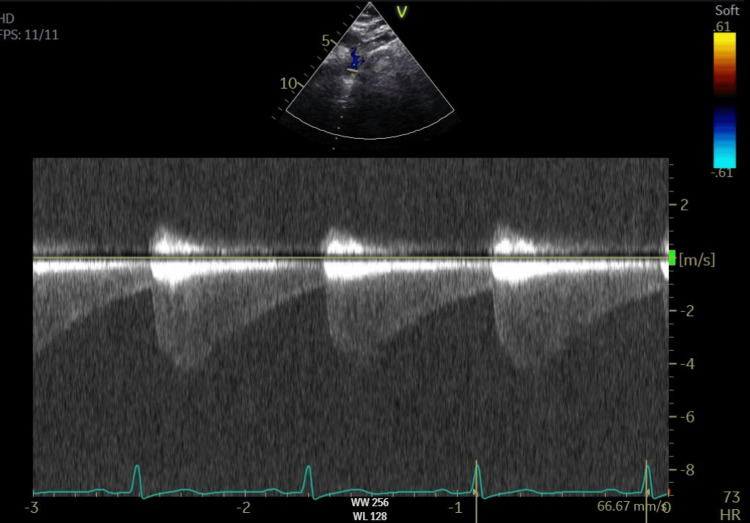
Repeat echo Suprasternal view of the aortic arch - continuous wave Doppler through the aortic isthmus with high-velocity systolic amplitude and continuous antegrade flow through diastole consistent with severe coarctation of the aorta. The peak pressure gradient was 78 mmHg, with a mean gradient of 40 mmHg.
